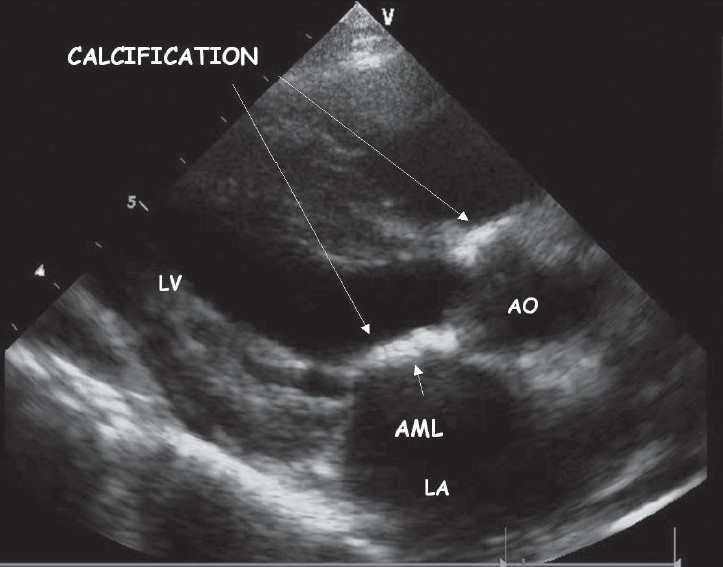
Parasternal long axis view in 2D echocardiogram showing calcification of the aortic root and the anterior leaflet of the mitral valve
